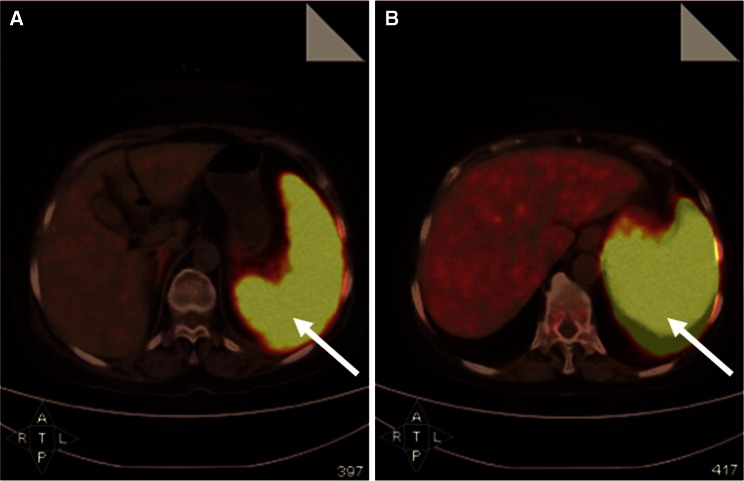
Comparison of PET–CT images of patient no. 15 before and after immunosuppressive therapy. a Initial PET–CT scan revealed FDG-avid splenomegaly with a SUV_Sp_ of 8.6 (*arrow*). b After receiving immunosuppressive therapy, post-therapeutic PET–CT revealed slightly smaller size in spleen; however, diffuse intense FDG uptake was higher than before with a SUV_Sp_ of 15.6 (*arrow*). In addition, new patchy FDG-avid lesions in humeri were shown, suggesting poor efficacy (not shown here). This patient survived for only 27 days
